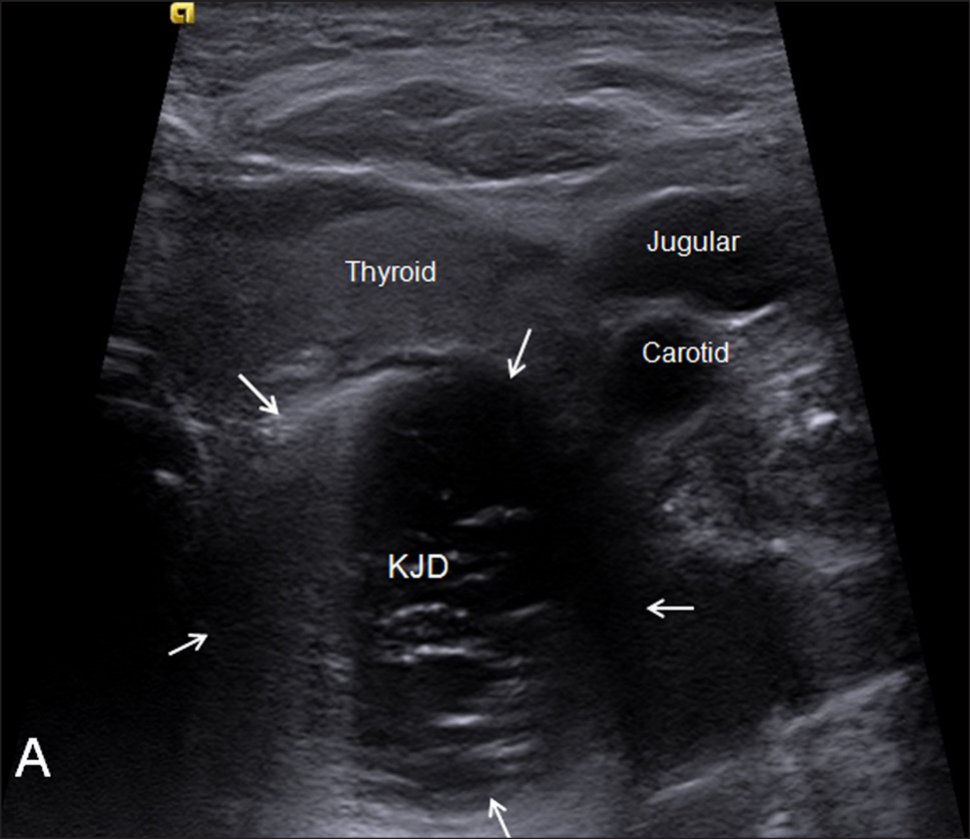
Ultrasonographic axial section showing KJD behind the thyroid left lobe.
